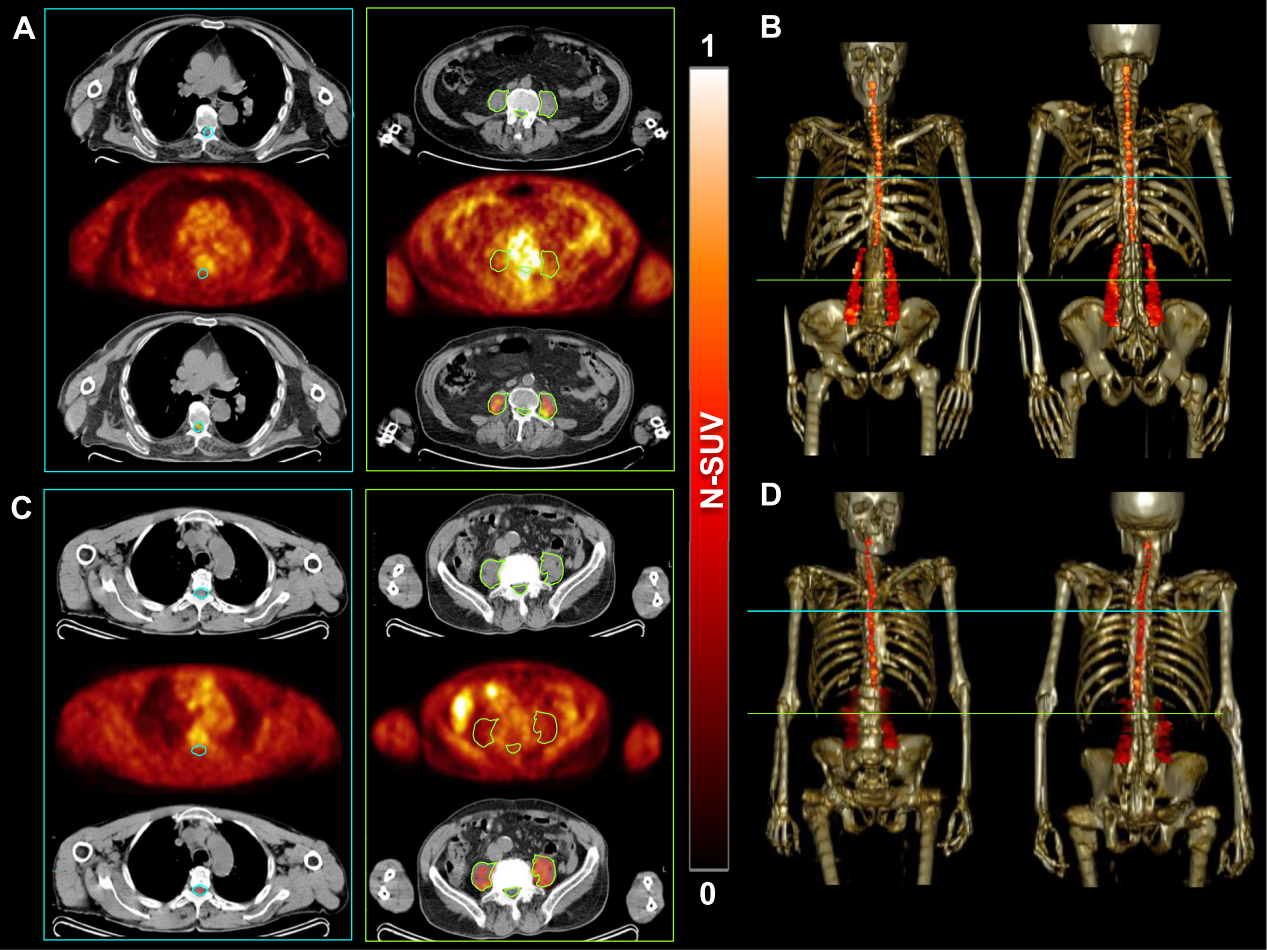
Examples of psoas muscle extraction from PET/CT images. Computational extraction of the spinal cord and psoas regions of interest from axial FDG PET/CT images in ALS patient (a) and healthy control (c). In panels b and d, the 3D reconstruction of obtained volumes of interest on the skeleton of these subjects are reported
